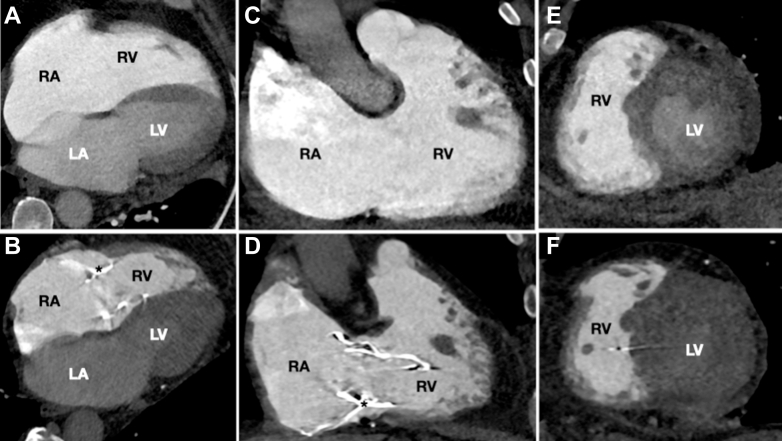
Cardiac CT Scan Before and After the Procedure Cardiac CT scans at baseline (A, C, and E) and at 1-year follow-up (B, D, and F) after orthotopic transcatheter tricuspid valve replacement (TTVR) using the Lux-Valve Plus system, demonstrating significant RV and RA reverse remodeling. The maximal RV basal diameter decreased from 54 mm to 41 mm, and RA area was reduced from 50 to 32 cm^2^. (A and B) Four-chamber views; (C and D) two-chamber views; and (E and F) transverse views. LA = left atrium; LV = left ventricle; RA= right atrium; RV = right ventricle.
